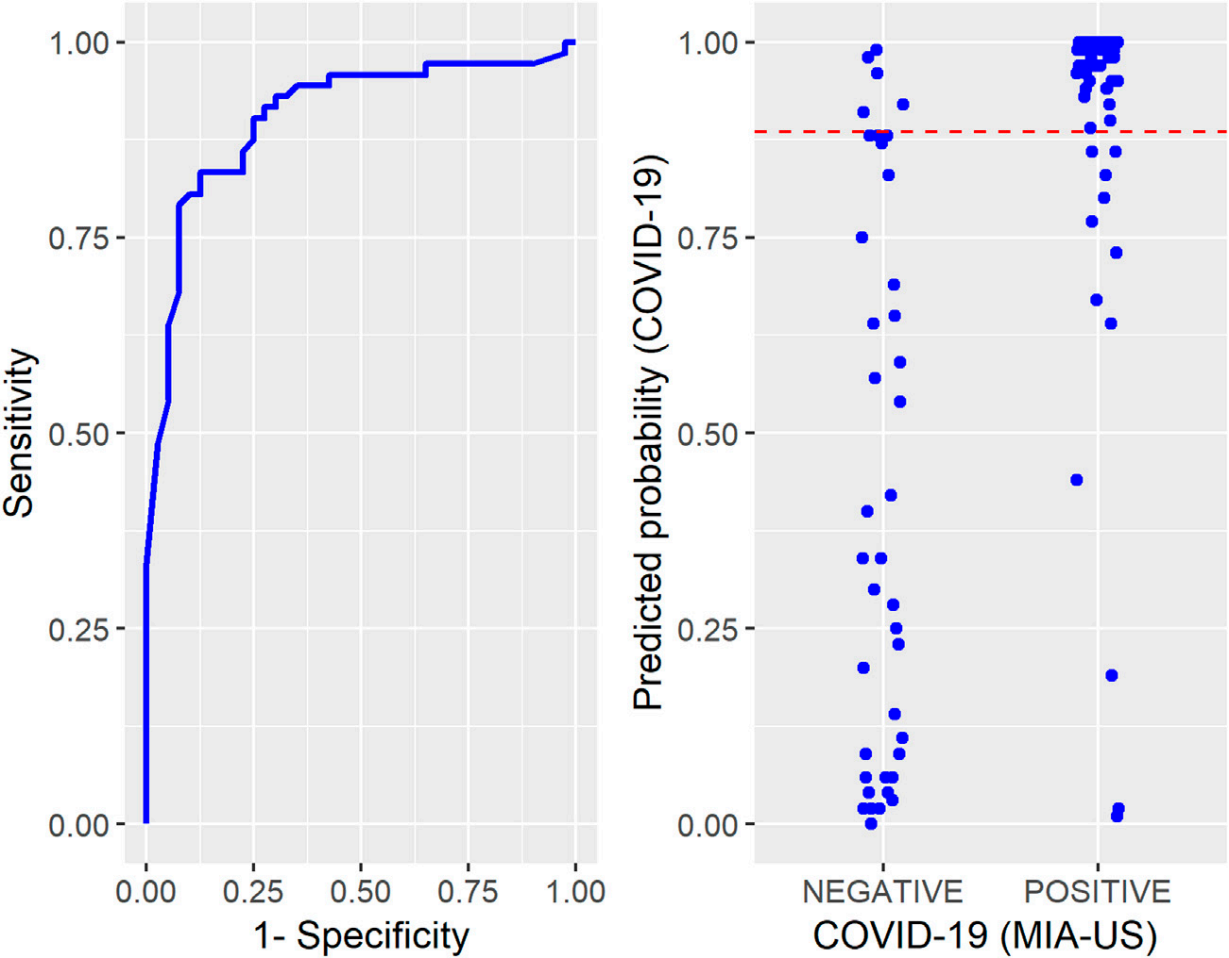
Left: Receiver Operating Characteristic Curve constructed to determine the cutoff for the probabilities of COVID-19 predicted by the InterVA CRMS algorithm. Right: Individual values of the predicted probabilities of COVID-19 according to the Ultrasound-guided Minimally Invasive Autopsy classification (positive or negative). The dashed line in red represents the cutoff - COVID-19 Case-Control study in Brazil project, Sao Paulo, Brazil, March - December 2020.
